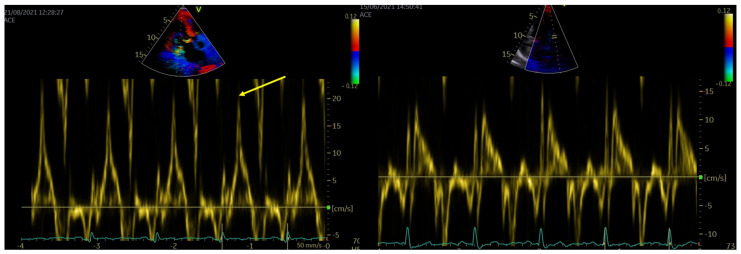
The presentation of the Pickelhaube sign (yellow arrow) as a spiked configuration of basolateral annular tissue Doppler imaging presentation (left panel). The presentation of S′ value of basolateral annular tissue Doppler imaging in patient without Pickelhaube sign (right panel).
